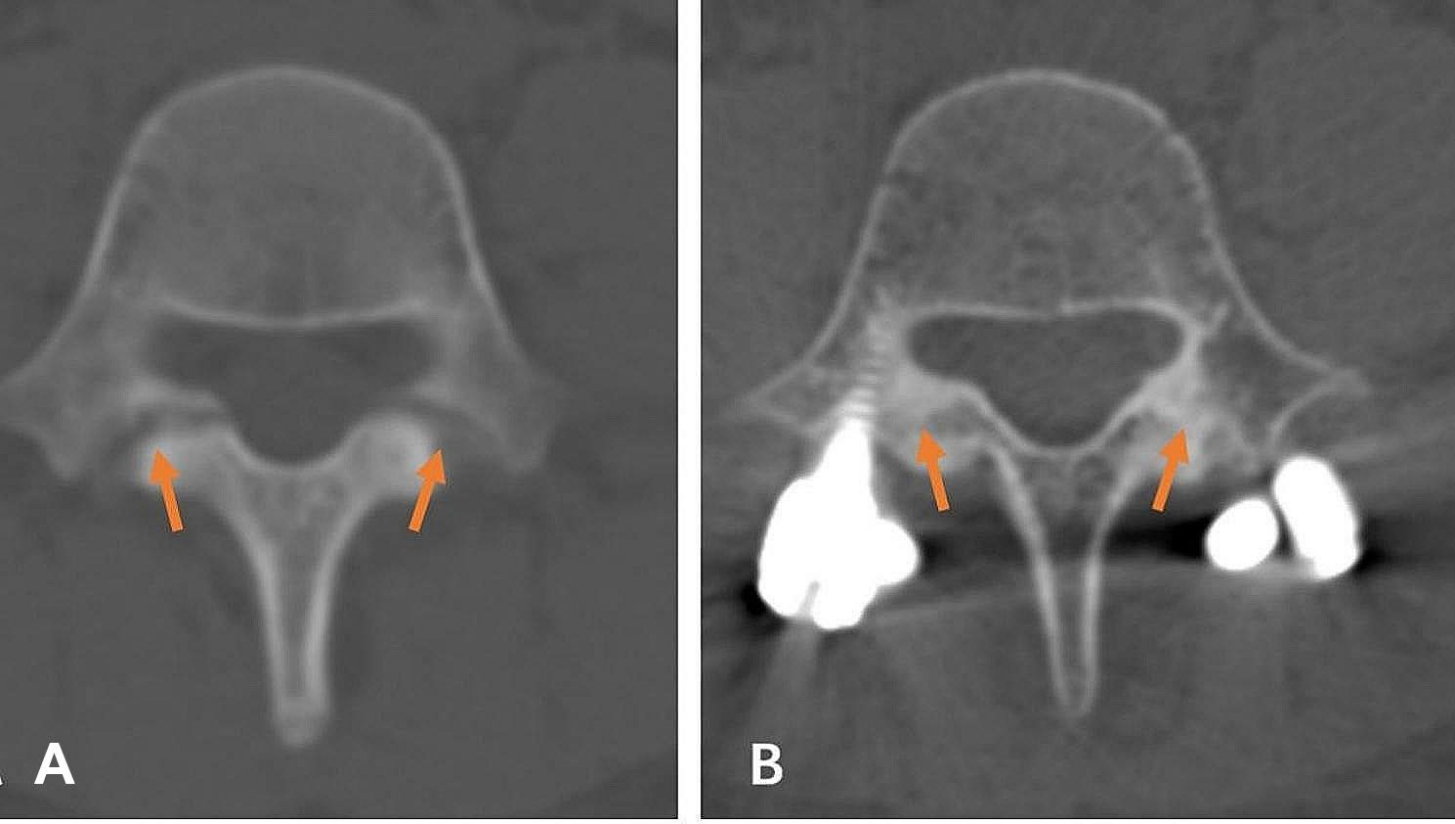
Representative axial CT images of successful fusion of the pars interarticularis defect. Pre-operative image of a patient with stage II pars defects (A). CT showing bilateral fusion at 14 months after pars repair surgery (B)
